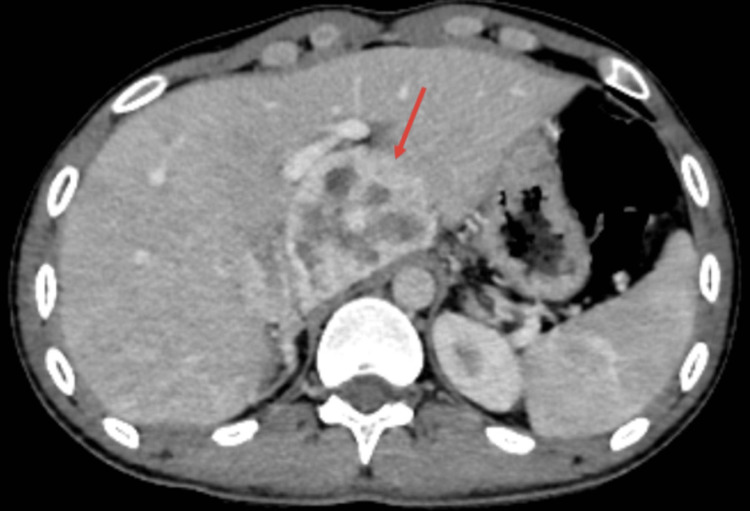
Cross section of CT scan imaging showing a tissular mass of the right adrenal gland CT, computed tomography.
